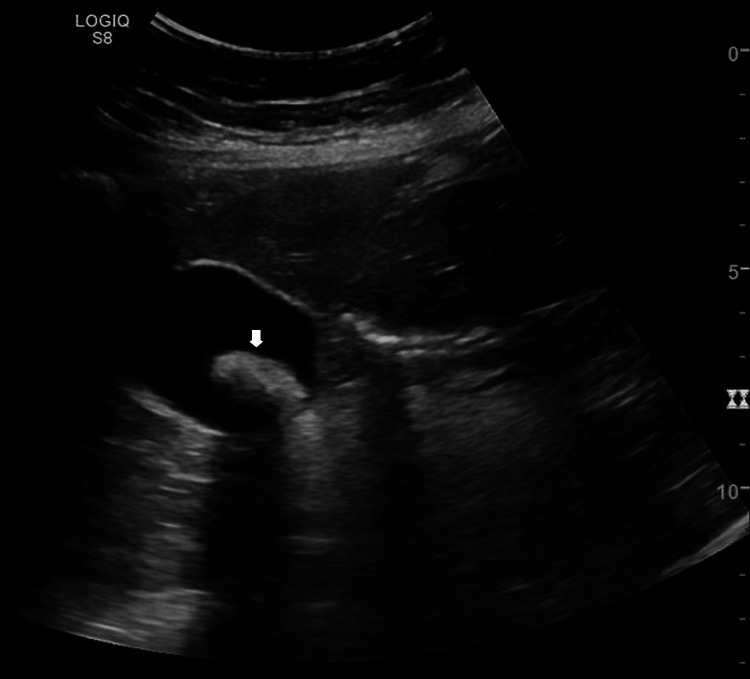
Abdominal ultrasound showing a gallstone (arrowhead)
